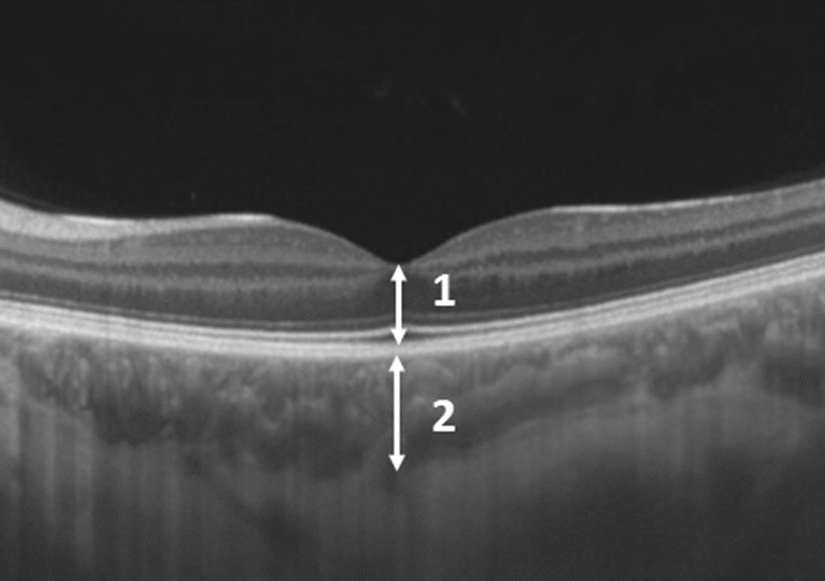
Choroidal (CT) and retinal (RT) thickness measurements. The features measured at the fovea included: (1) the CT (from the outer border of the retinal pigment epithelium to the inner scleral border), and (2) the RT (from the internal limiting membrane to the Bruch’s membrane)
